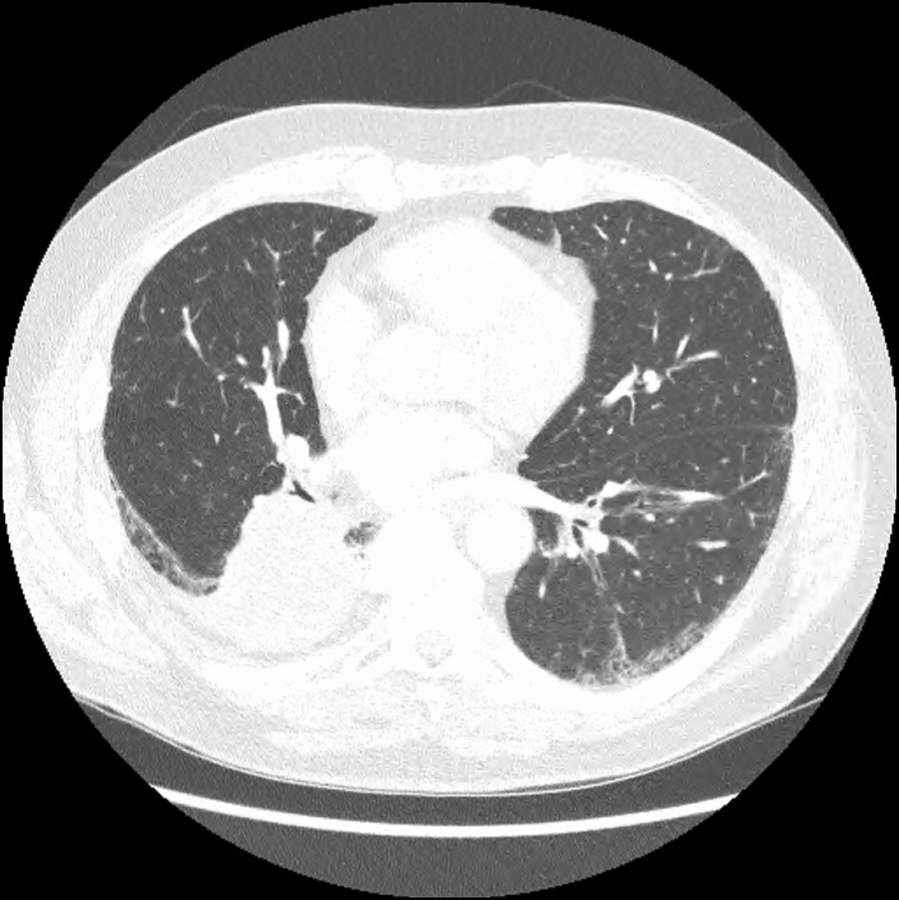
Case 2—CT chest upper lobes revealing signs of pneumonitis, widespread mixed ground glass and interstitial infiltrate. There is progression of malignancy
